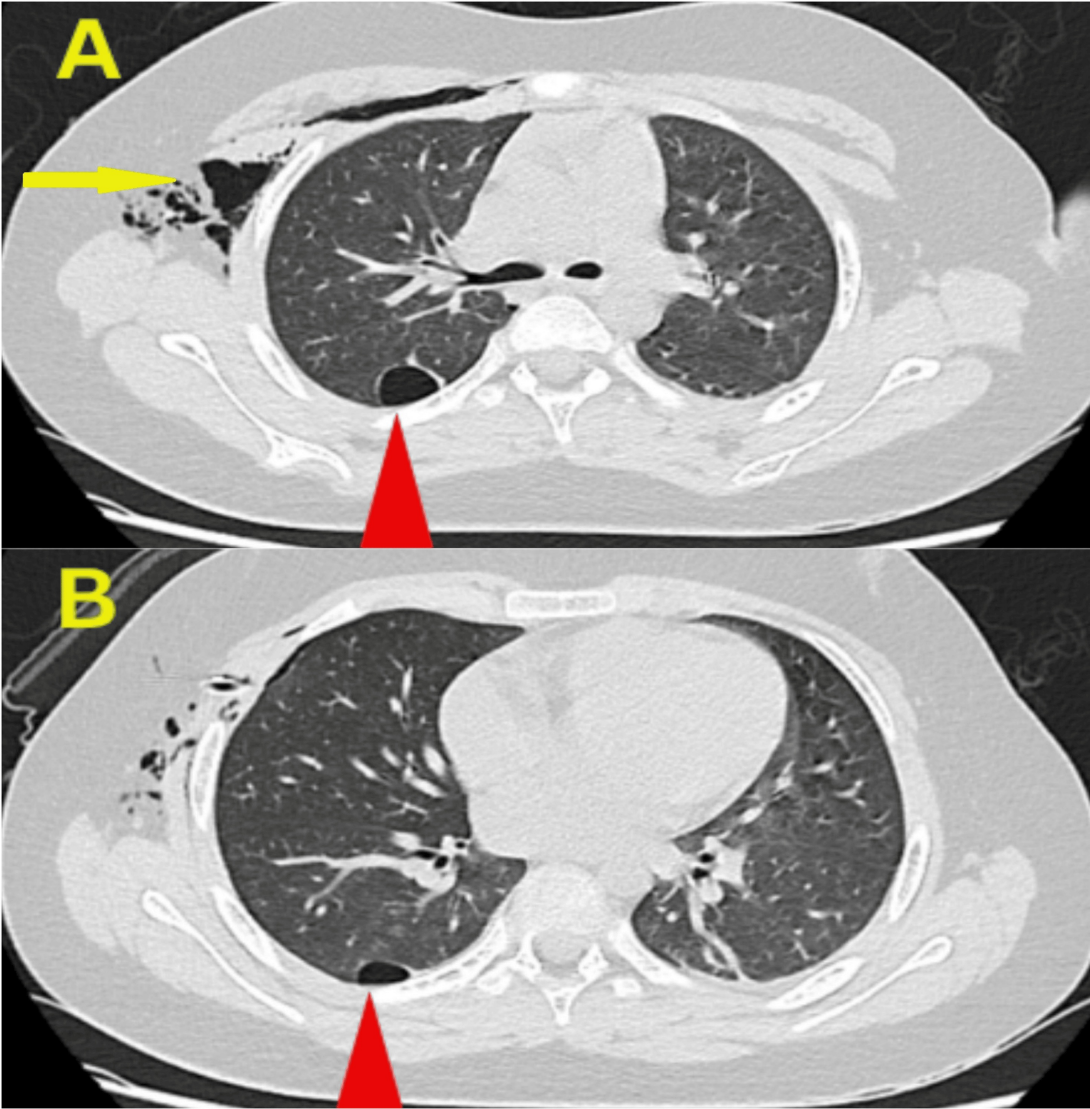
Post-drainage chest CT (A) Peripheral cystic lesion with a thin wall in the apical segment of the right lower lobe, consistent with a bulla (red arrow), along with post-drainage subcutaneous emphysema (yellow arrow).
